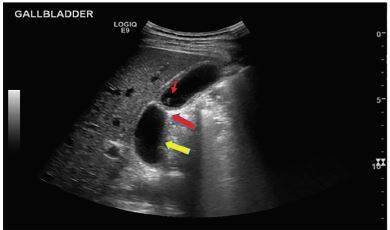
Ultrasound showing a Phrygian cap (large red arrow) and pseudo-duplication (large yellow arrow) with mild gallbladder wall thickening (measuring 3.3 millimeters) and gallstones (small arrow).
